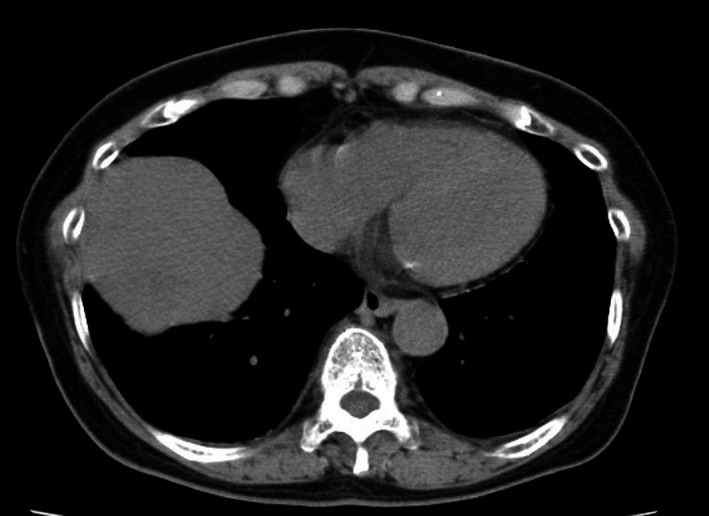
Computed tomography showed a homogenous, sharply defined mass, which was in contact with the middle lobe.
